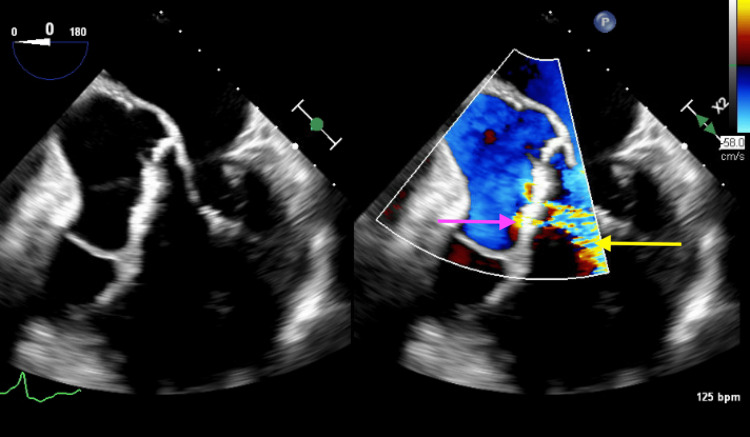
Transesophageal echocardiogram demonstrating perforation of the right cusp (pink arrow) with associated aortic regurgitation (yellow arrow).
